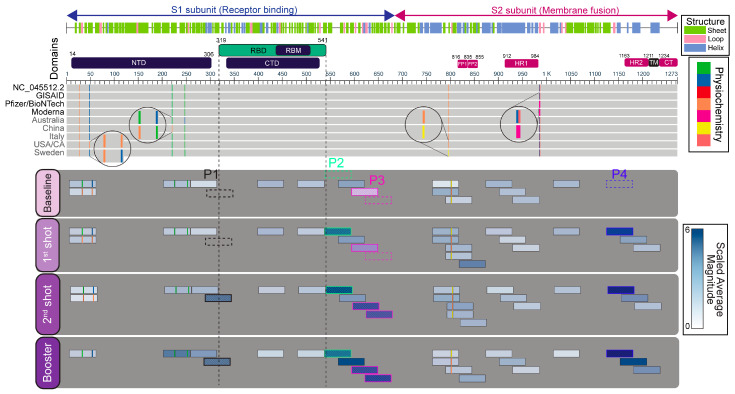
Epitope map of anti-S responses represented as scaled average magnitudes. The multiple sequence alignment (MSA) of full-length SARS-CoV-2 spike glycoprotein reference, vaccine, and input library sequences are shown, where regions in light grey represent sequence conservation. Mismatched residues are highlighted and color-coded using the pre-defined Zappo scheme (green: hydrophilic, salmon: aliphatic/hydrophobic, orange: aromatic, fuchsia: conformationally special, yellow: cysteine only, red: negatively charged, blue: positively charged). Reactive peptides are mapped and colored based on scaled average magnitudes (blue gradient) across timepoints. Vaccine-boosted epitopes are indicated (P1–P4). Peptides with transparent backgrounds and dashed borders indicate absence of baseline responses and are only shown for convenience. Abbreviations: NTD: N-terminal domain, CTD: C-terminal domain, RBD: Receptor-binding domain, RBM: Receptor-binding motif, FP1/2: Fusion peptide 1/2, HR1/2: Heptad repeat 1/2, TM: Transmembrane, CT: Cytoplasmic tail.
